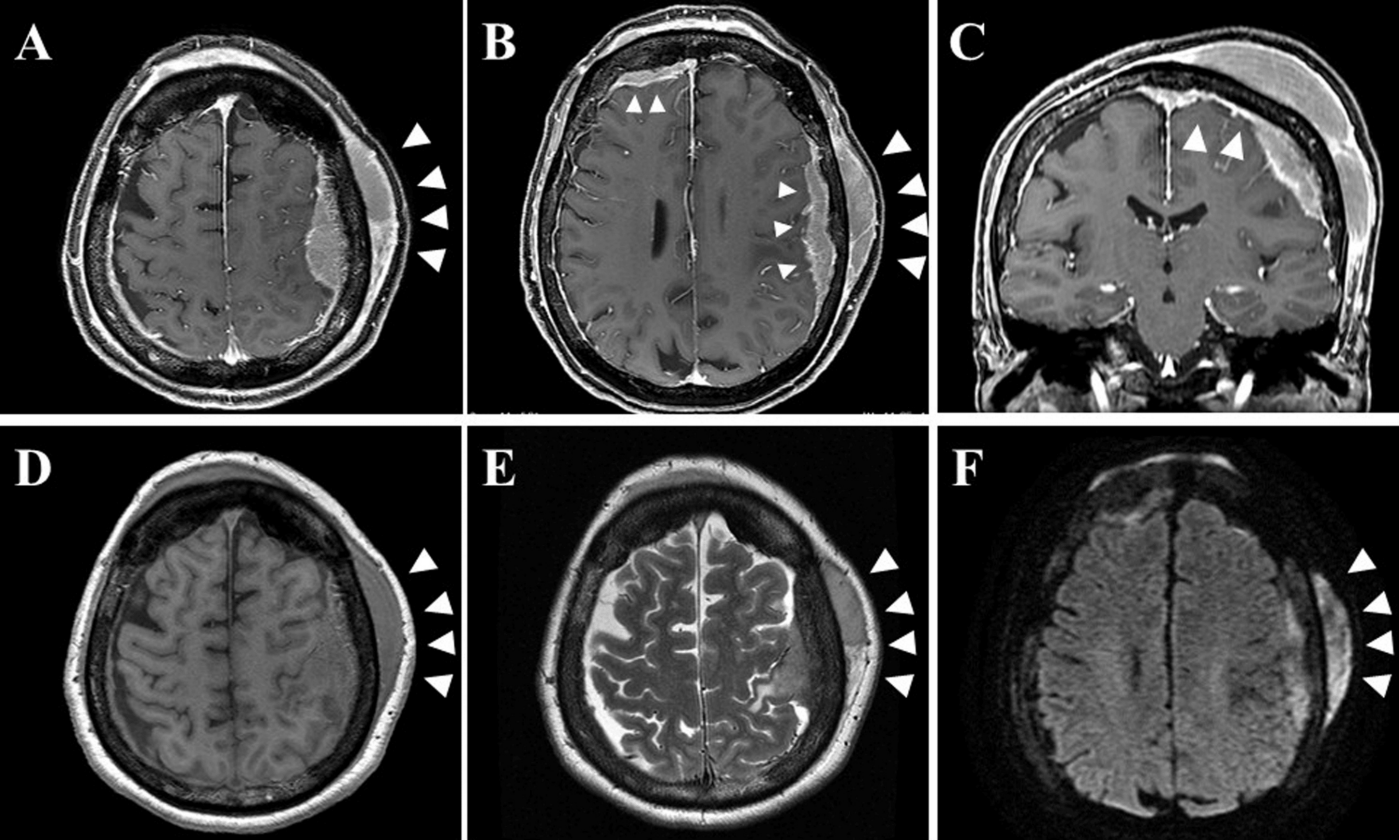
Magnetic resonance images (MRI) Axial contrast-enhanced T1-weighted image showing a mass lesion with extensive intracranial and extracranial enhancement (arrowhead) (A). Axial image showing a dural lesion extending continuously from the right frontal to left parietal region (arrowhead) (B). Coronal image showing dural tail sign (arrowhead) (C). Axial T1-weighted image showing an isointense lesion (D). Axial T2-weighted image showing the lesion as slightly hyperintense (E). Diffusion-weighted image showing the lesion as hyperintense (F).
